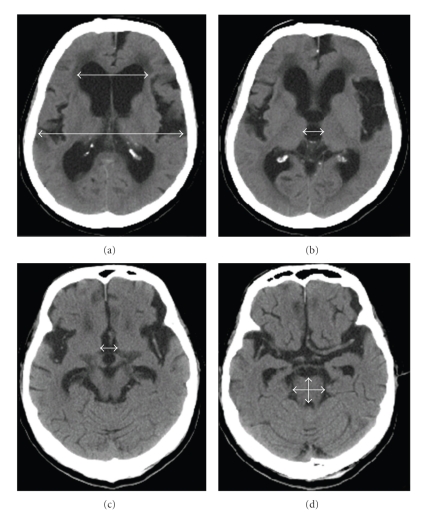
Measurements for iNPH patients on CT scans. (a) Evans index (ratio of maximum width of the frontal horns to the maximum width of the inner table of the cranium); (b) maximum width of the third ventricle at the foramen of Monro (interventricular foramen); (c) maximum width of the third ventricle at the superior colliculus; (d) anteroposterior (vertical) and left-to-right (horizontal) diameters of the midbrain at pontomesencephalic junction.
